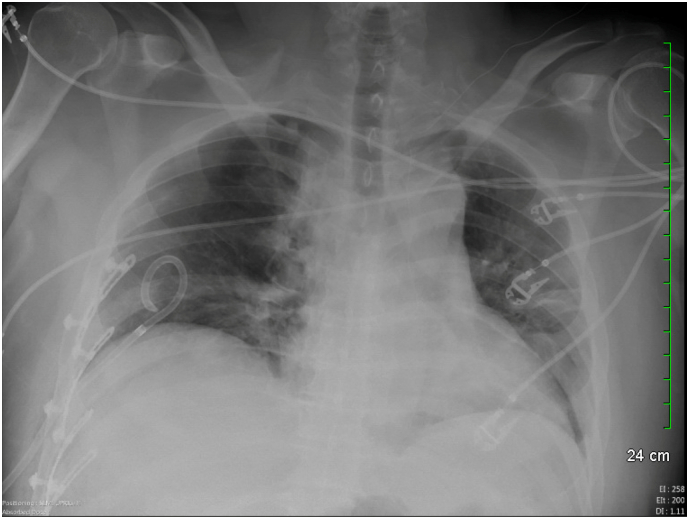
Chest X-ray showing intrathoracic rib plates on right ribs 6 through 9.
